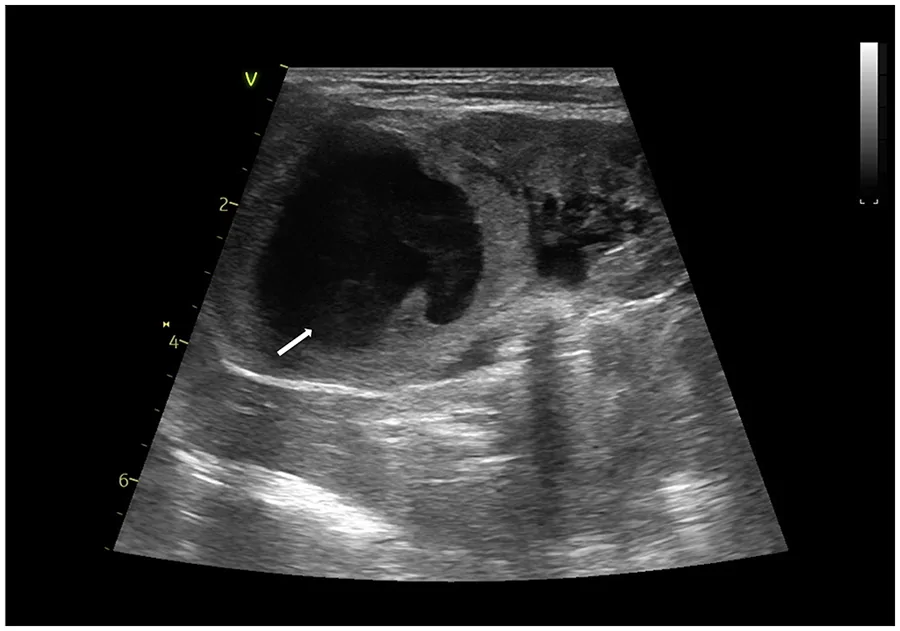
Ultrasound image demonstrating a large right-sided suprarenal mass with internal hypoechoic areas (arrow).
